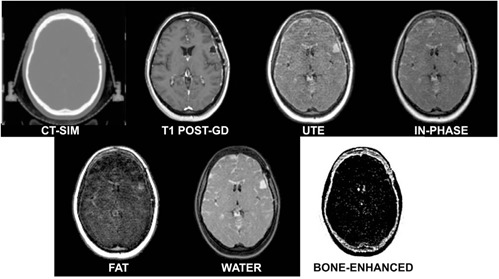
Axial CT‐SIM and 1.0T MR‐SIM images for a postsurgical stereotactic radiosurgery patient with a left frontal metastasis, including T1 post‐Gd, ultrashort echotime (UTE), water and fat images generated via Dixon acquisitions, and a bone‐enhanced image derived from the in‐phase and UTE datasets.
